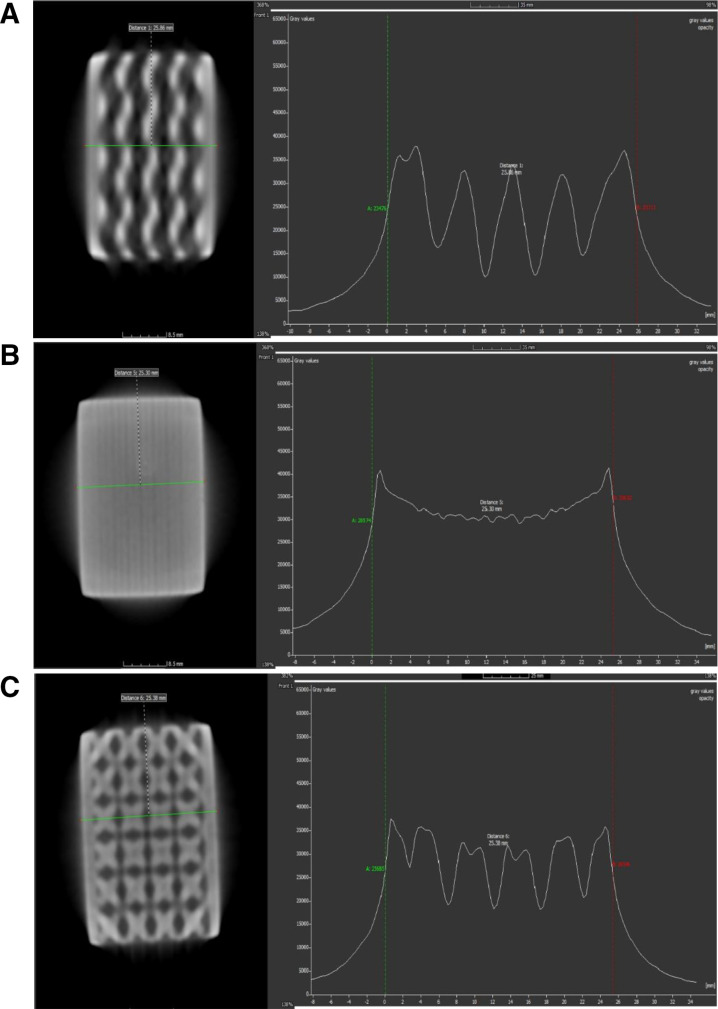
(a) CT scan analysis of gyroid lattice with nodal density distribution. (b) CT scan analysis of honeycomb lattice with nodal density distribution. (c) CT Scan analysis of BCC lattice with nodal density distribution.
